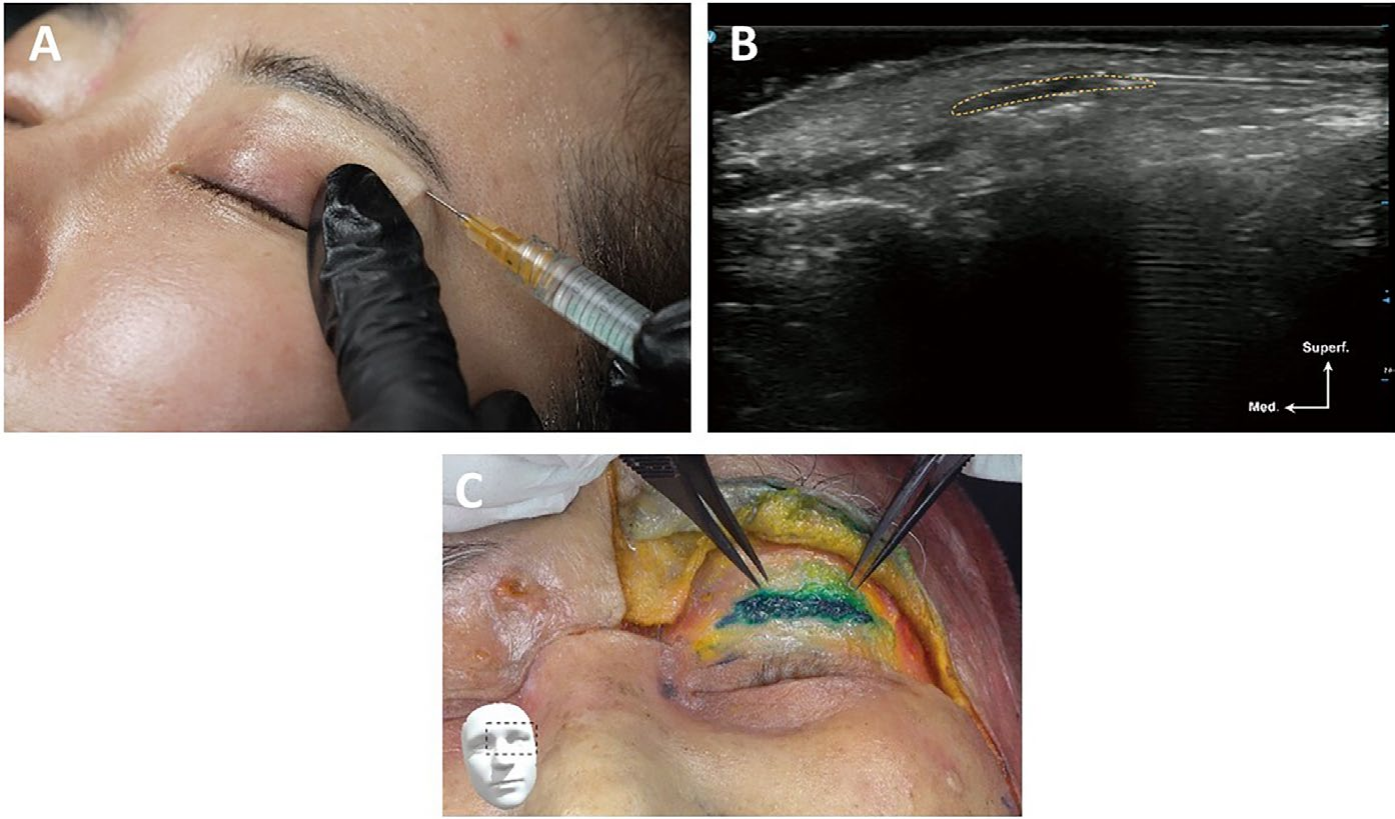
A demonstration of superficial plane injection of filler in the upper eyelid (41‐year‐old Korean female). (A) Clinical injection. (B) Clinical ultrasound. (C) Cadaver dissection (72‐year‐old Korean male).
